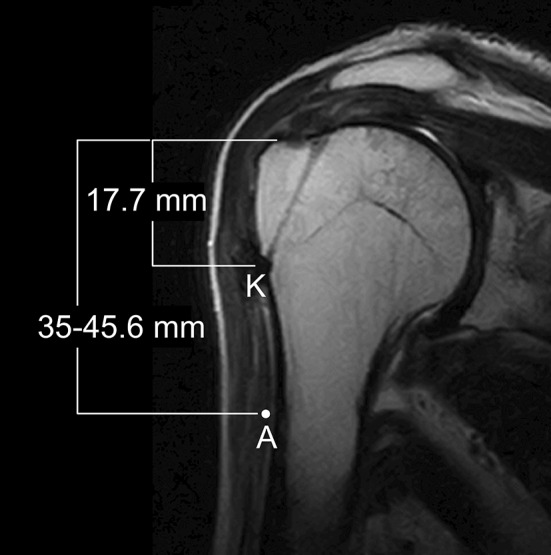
A postoperative MR image shows the average distance from the superior border of the greater tuberosity to the insertion point of the K-wires (K) as 17.7 mm. The distance between the superior border of the greater tuberosity and the axillary nerve (A) has been reported as 35 to 45.6 mm.
